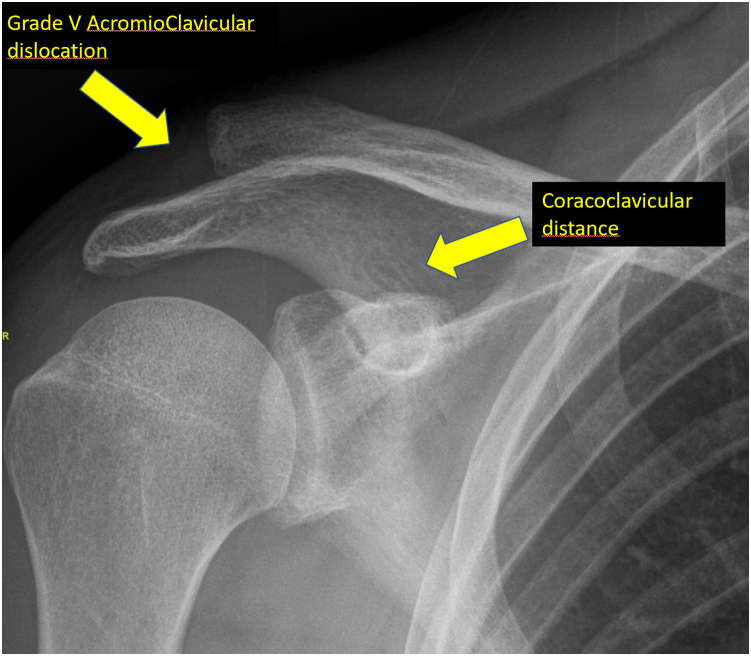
Preoperative radiograph of a patient with grade V acromioclavicular dislocation. There is no contact between the acromion and the clavicle and a large coracoclavicular distance.
